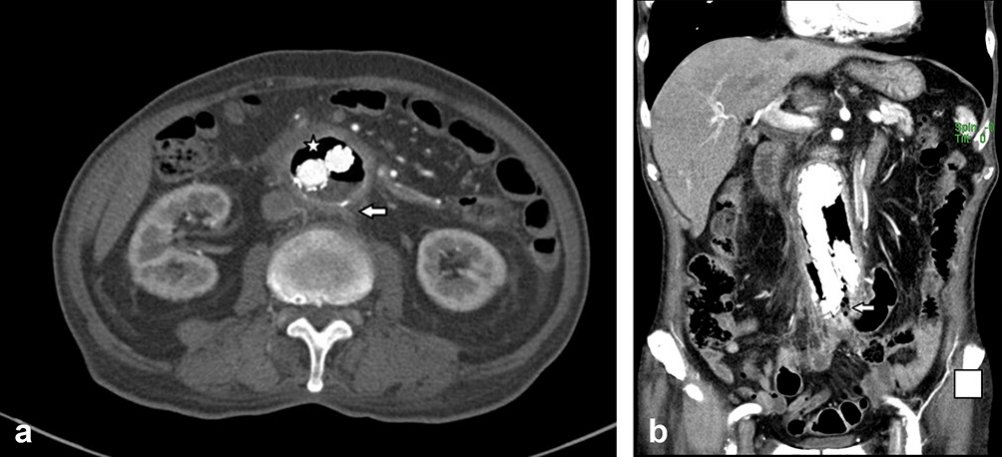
Contrast-enhanced CT images in axial (a) and coronal (b) sections: a—air is noted surrounding the endovascular graft (star), with aortic wall inflammation (arrow); b—air with multiple air pockets seen surrounding the endovascular graft, extending to the level of the common iliac arteries (arrow).
